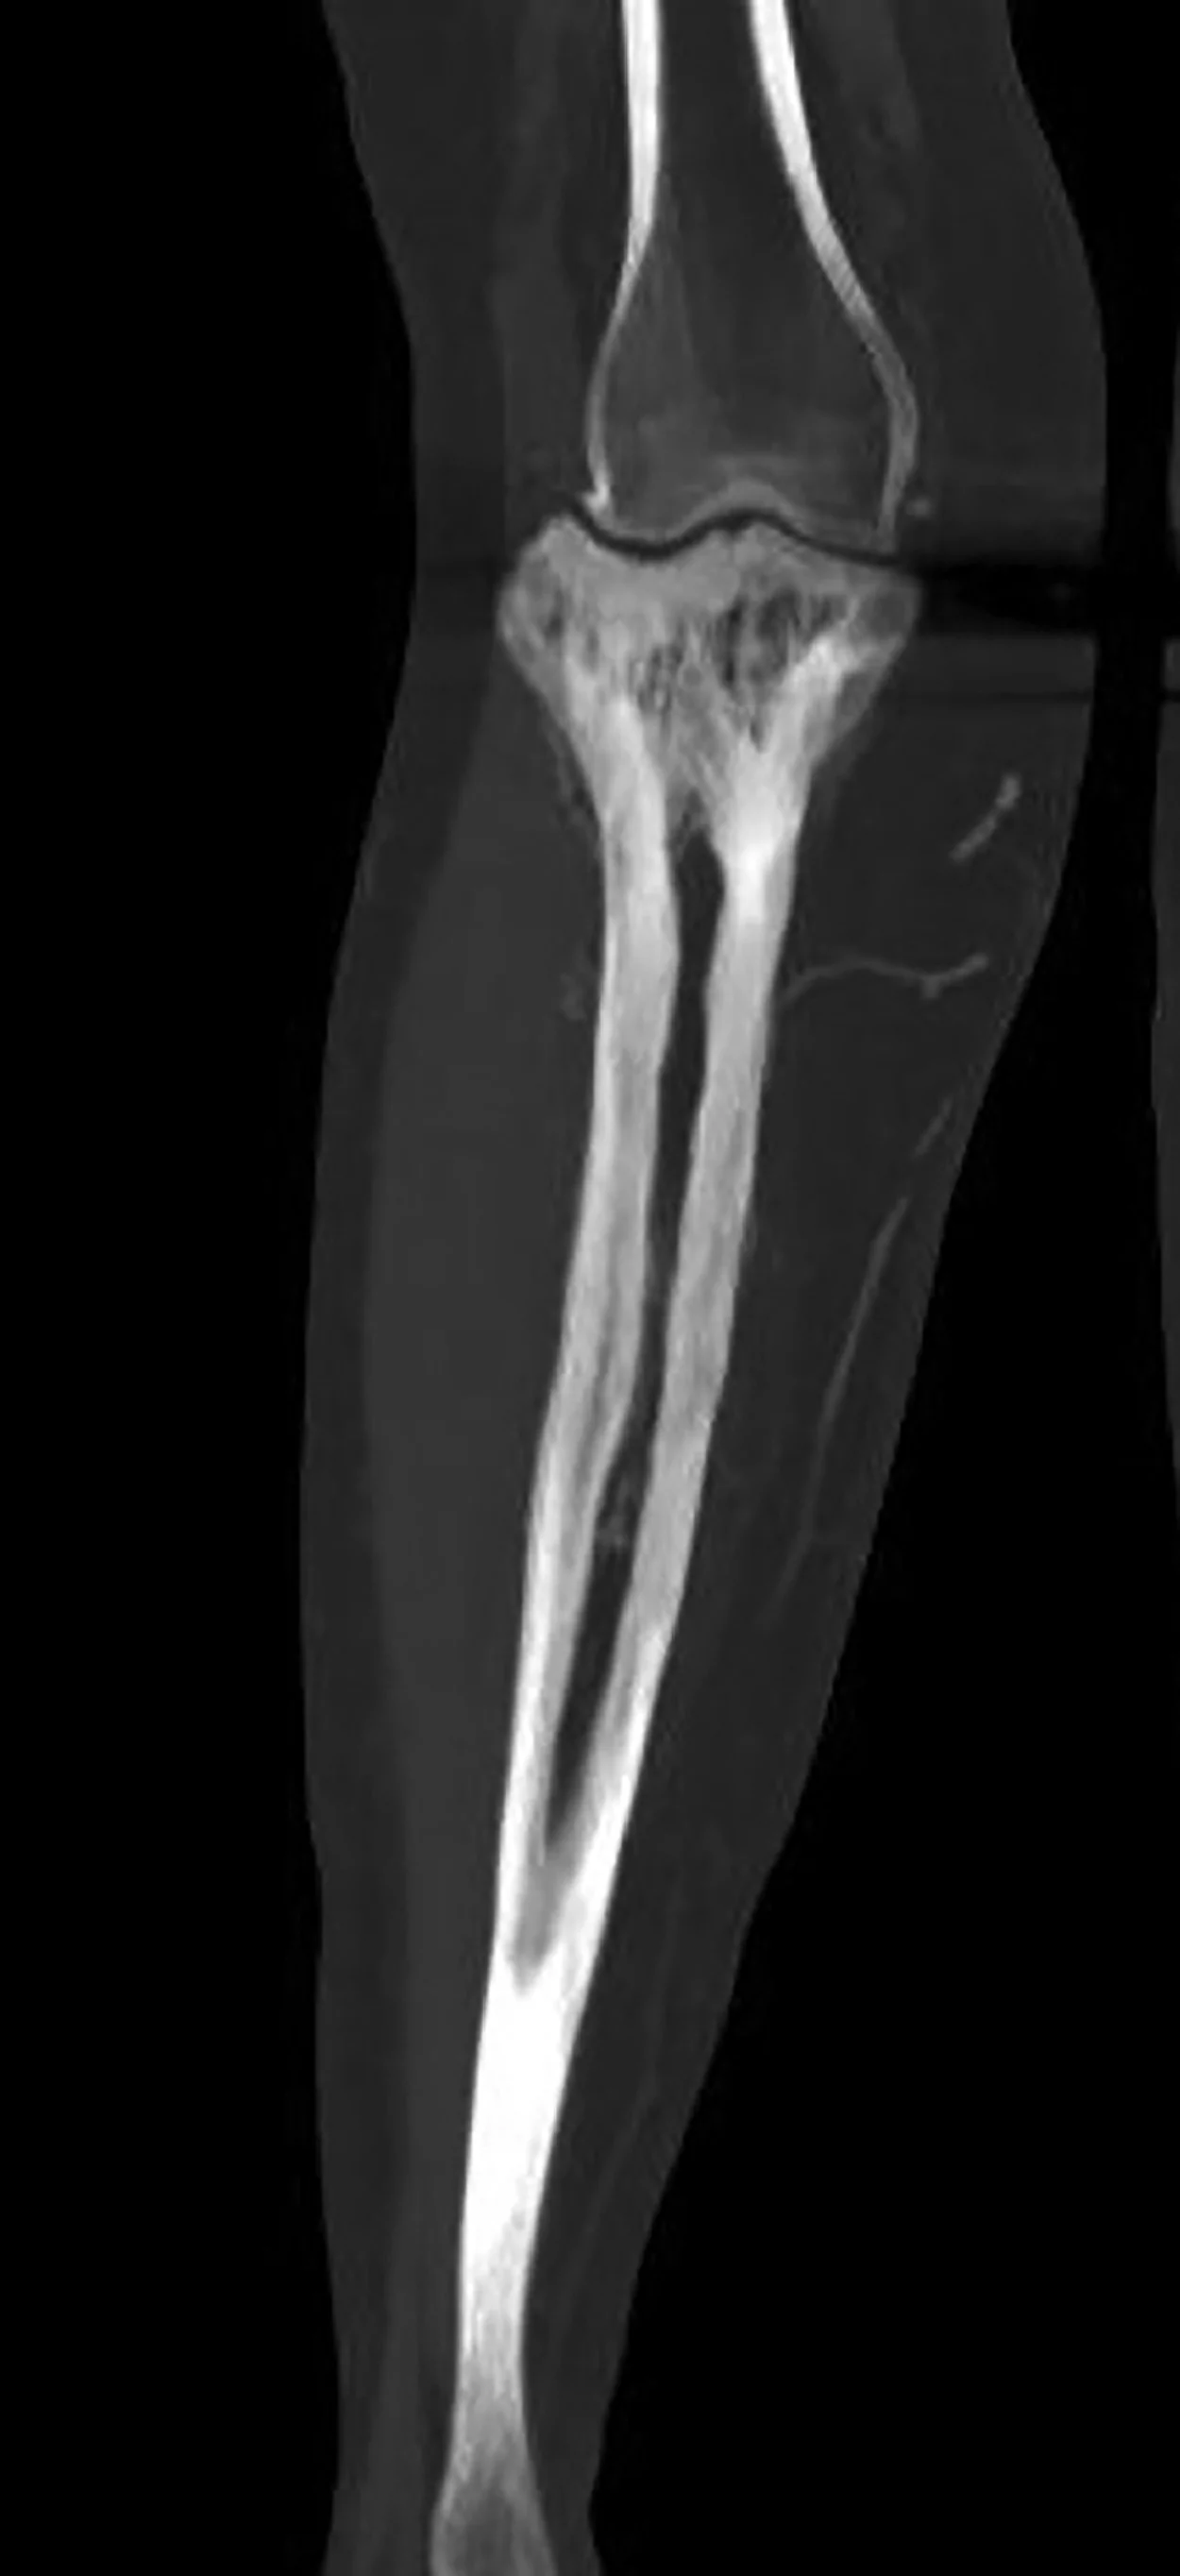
Coronal CT image confirms cortical and trabecular thickening of the right tibia, consistent with Paget’s disease of bone. No focal lucent lesion is seen.
